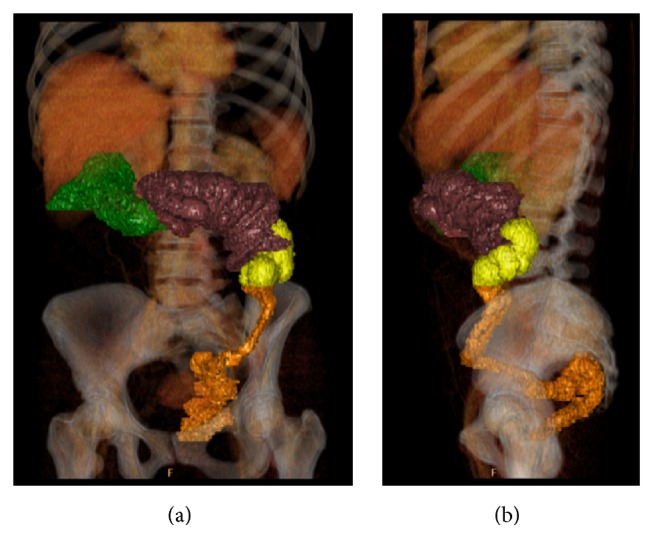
Contrast-enhanced CT, portal venous phase, 3D volume rendering reconstructions. The anatomical structures of interest were segmented for a panoramic view: the dilated last ileal loop (green), the intussuscepted colonic segments (purple-brown), the lipoma (yellow), the collapsed distal third of the left colon, and the sigmoid and rectum (orange). The skeletal structures and the parenchymatous organs were left in transparency.
